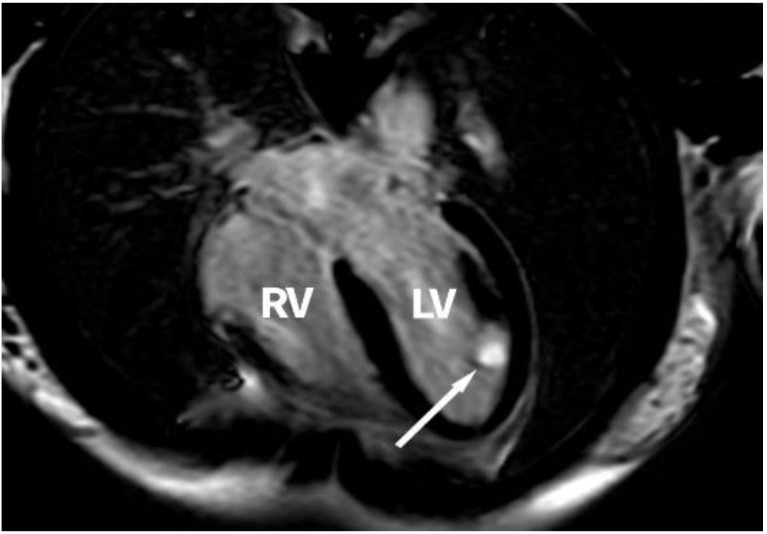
Cardiac magnetic resonance image of the tumour in the left ventricle and papillary muscle at the base of the anterolateral papillary muscle (white arrow, left ventricle tumour); RV, right ventricle; LV, left ventricle.
